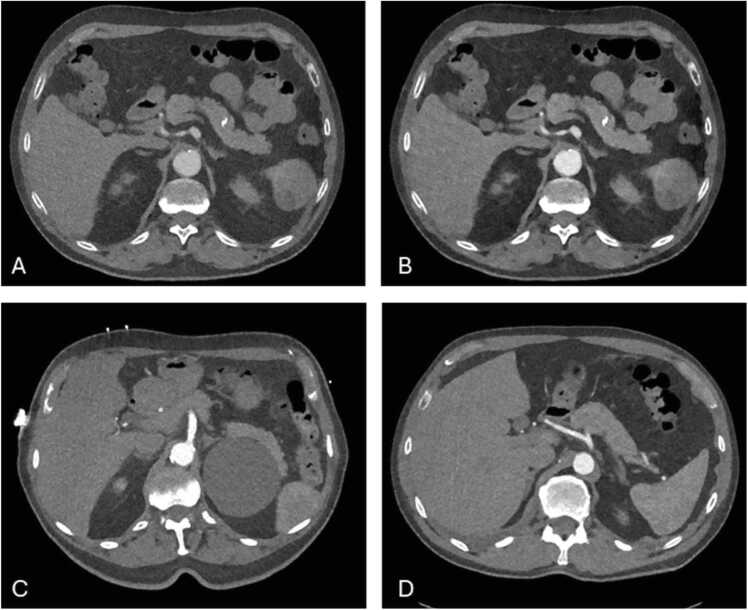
Photon-counting detector CT angiography in different patients with comparable body mass index. Images were generated with 55 keV ultra-low contrast agent dose (A), 45 keV ultra-low contrast protocol (B), standard contrast agent dose (C), and low contrast agent dose (D). Window settings: width 700 HU; center 100 HU.
